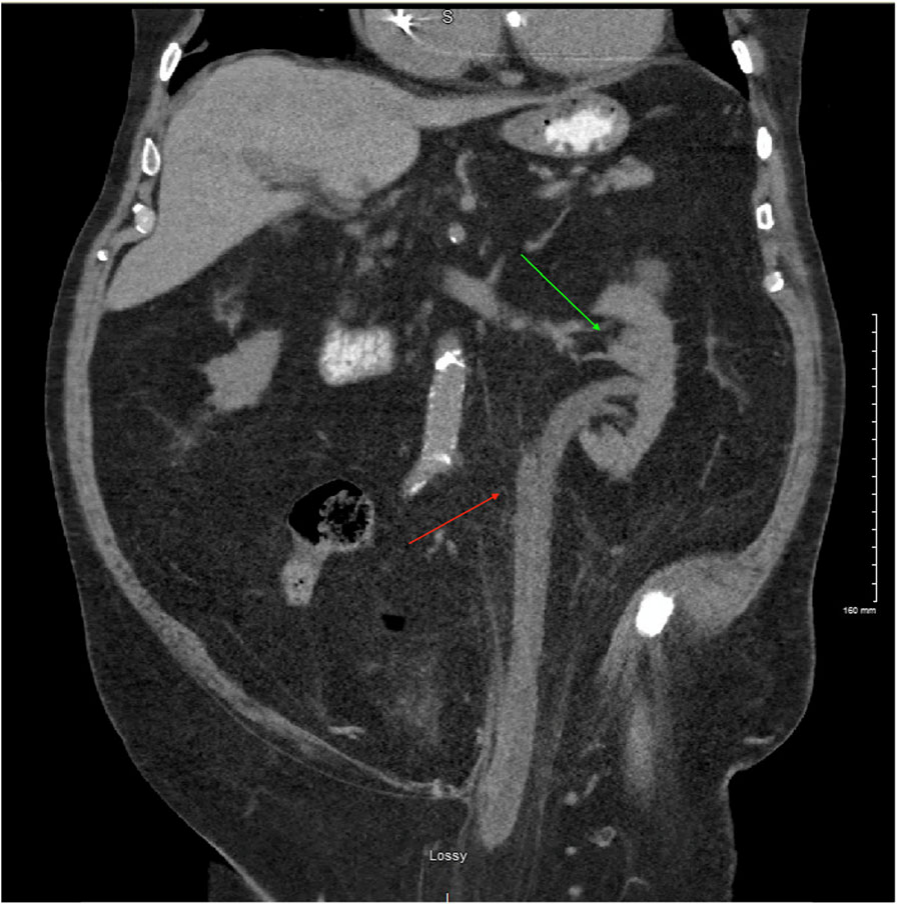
CT scan of the abdomen and pelvis showing the hydroureternephrosis. (Green arrow: Hydronephrosis, Red arrow: ureteronephrosis)
